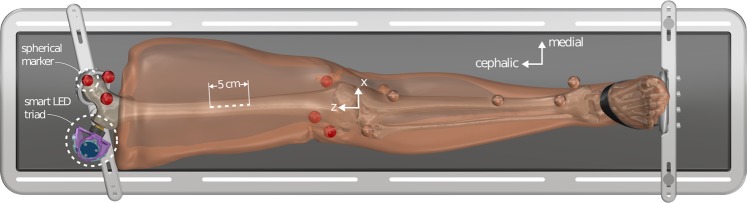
A leg model segmented from a CT scan depicted in the adjustable imaging platform. The spherical markers used in the current study are shown in red on the femur along with motion tracking marker (smart LED triad). Surgical acts were performed on central, anterior region of the upper leg with a target cut length of 5 cm. Femur coordinate system is also shown. The CT scan segmentation was developed from a related study^21^.
